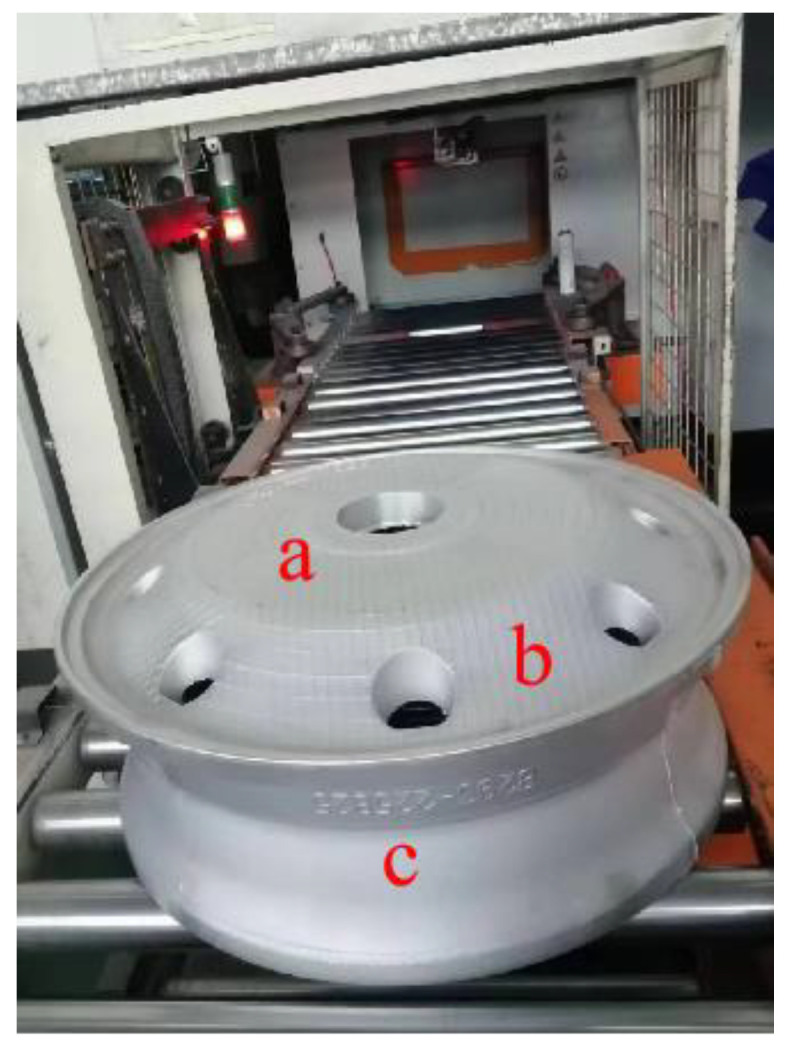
X-ray inspection location map.
